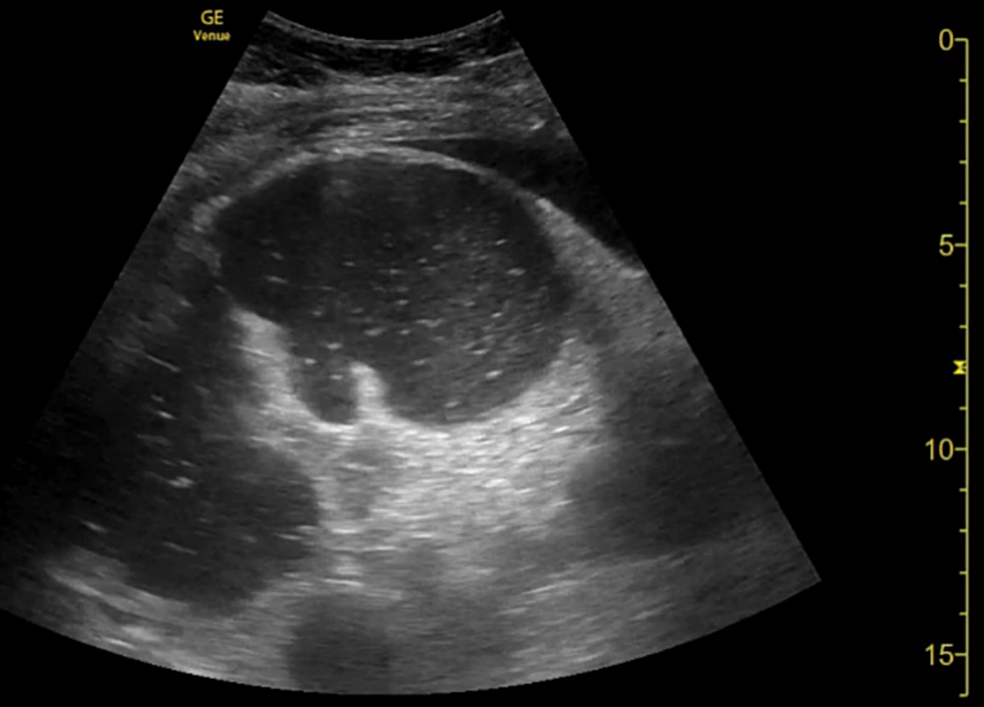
Point-of-care ultrasound showing dilated bowel loops in the right lower quadrant filled with anechoic secretions.
